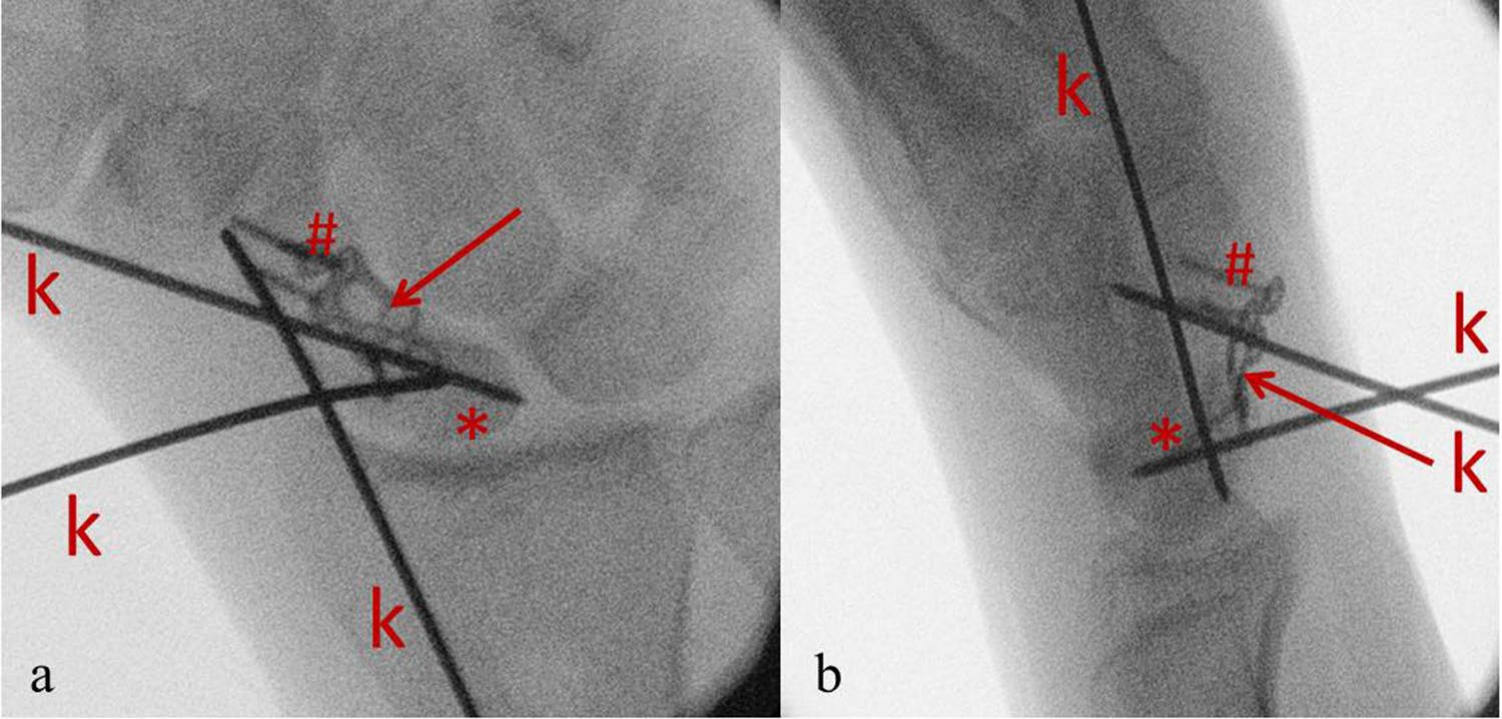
Intraoperative X-ray anteroposterior (a) and lateral (b) of scaphoid reposition and plate positioning. Using 2 K-wires (k) as joysticks, the proximal (*) and distal fragment (#) of the scaphoid are aligned and fixed with a third. The scaphoid plate (arrow) bridges the defect between the fragments via inserted angle-stable screws.
